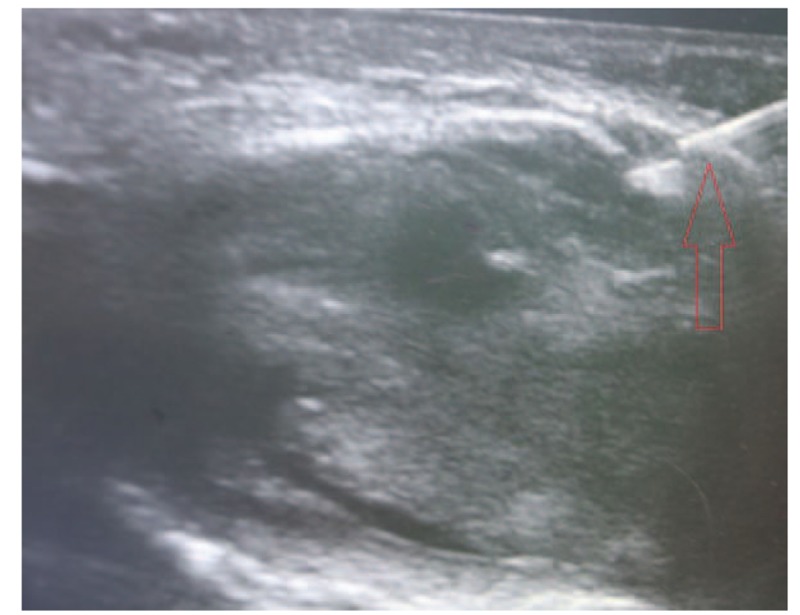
Aspiration biopsy guided by ultrasound.
